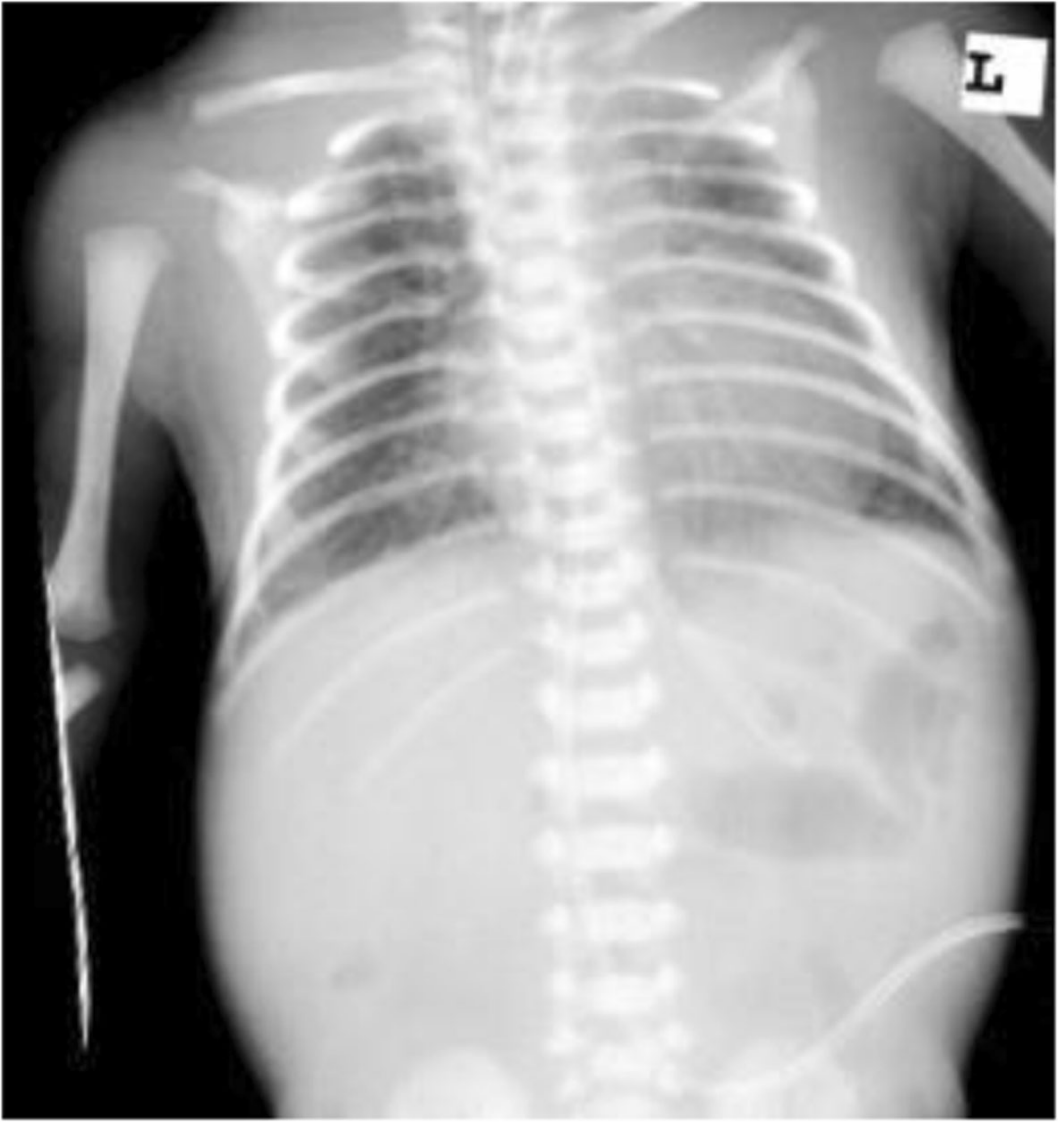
Chest x-ray of case 2. Image date: 2020/03/05
